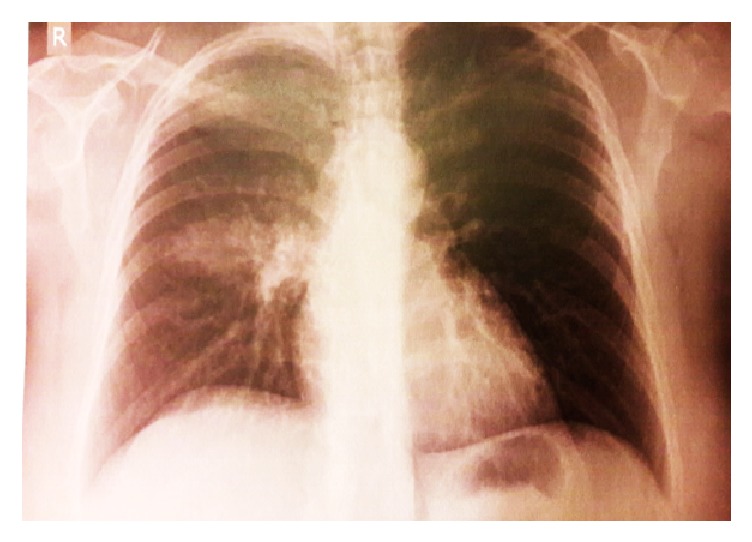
Patient's chest X-ray demonstrating a right upper lobe consolidation.
